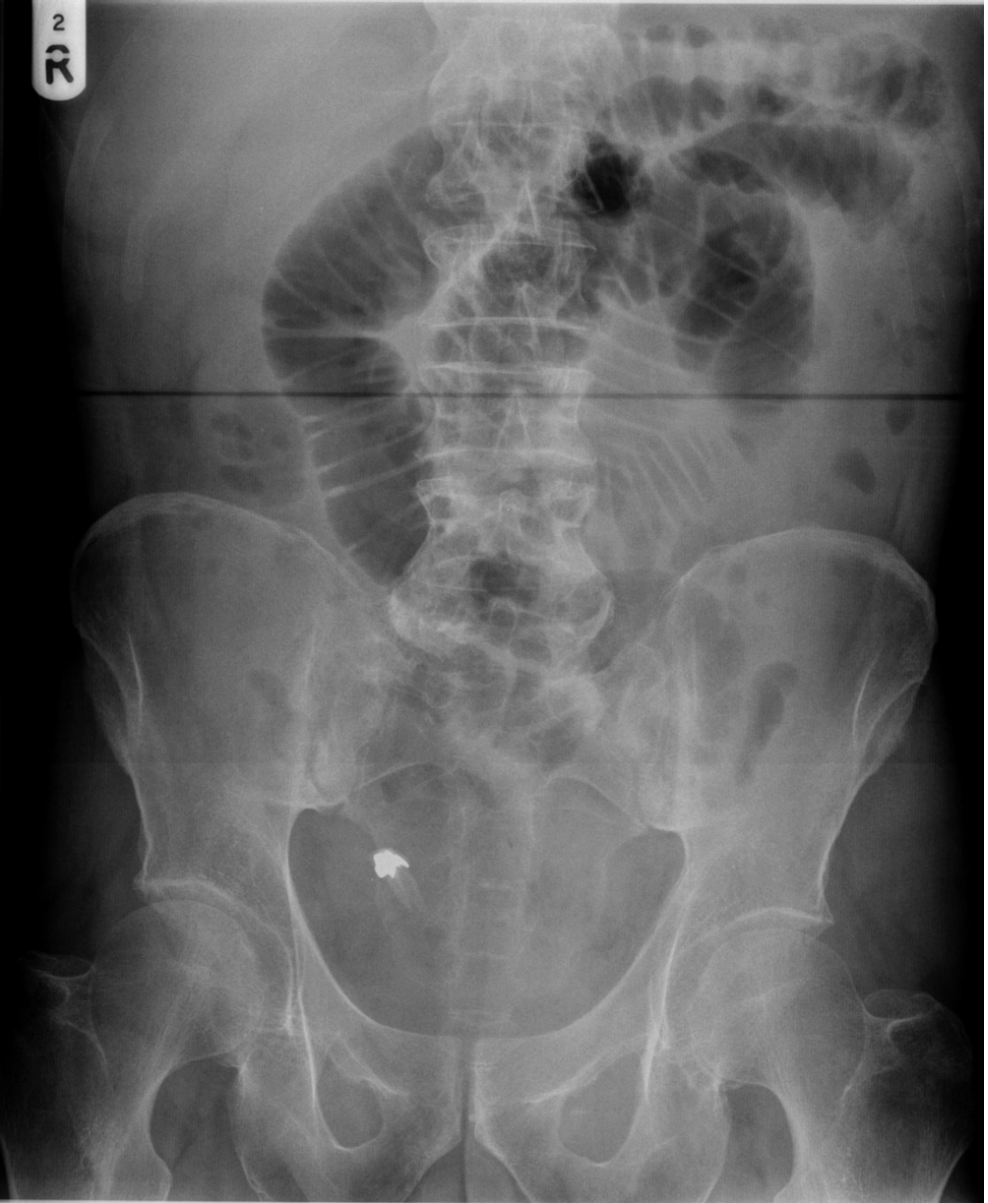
Abdominal x-ray showing denture causing small bowel obstruction.
